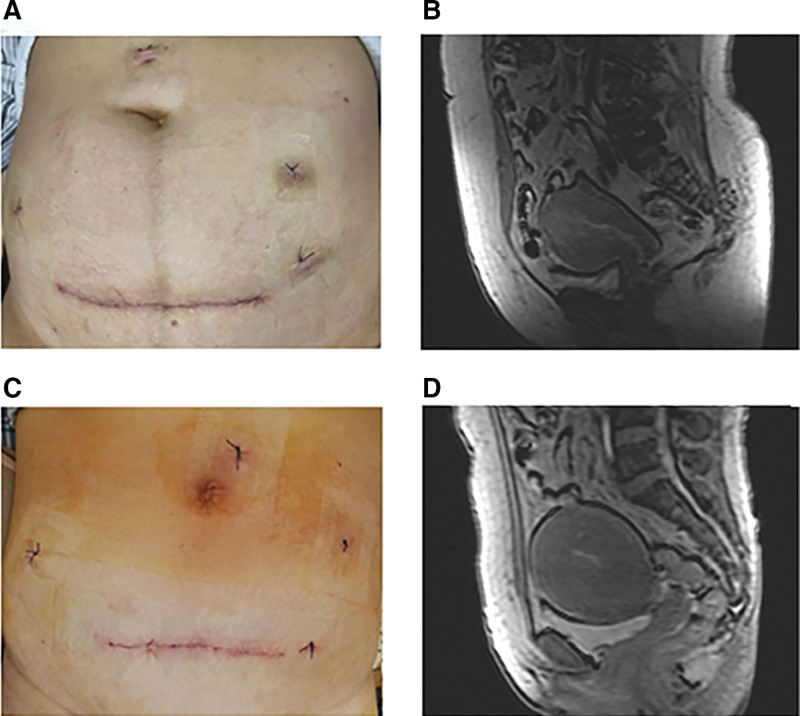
The incision and MRI image of case 1 (A and B) and case 2 (C and D) patients. (A) The incision of the lower abdomen of Case 1, (B) the size of her uterus was 7.3 × 8.0 × 7.6 cm and there was a 6.6*4.5*4.8 cm space-occupying shadow in uterine cavity myometrium, with unclear boundary, more than 1/2 the thickness of myometrium invasion in MRI image, (C) the incision of the lower abdomen of case 2, and (D) the size of her uterus was 8.5 × 8.9 × 8.5 cm and the tumor in uterine cavity infiltrate the superficial muscle layer in MRI image. MRI = magnetic resonance imaging.
